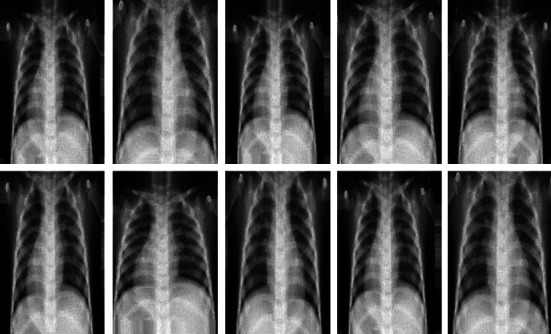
Variations of a chest X-ray image.
